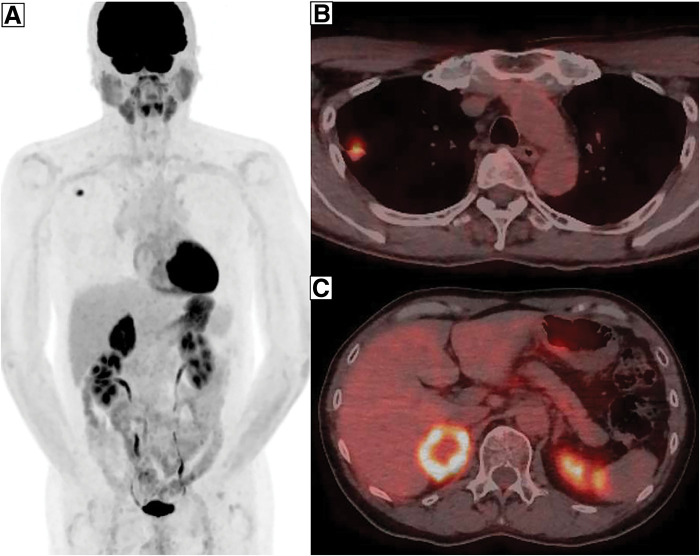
FDG-PET scans. FDG-PET scans show abnormal uptake (A) in the lung nodule (B, SUVmax = 7.2) and in the adrenal mass (C, SUVmax = 11.6). FDG, ^18^F-fluorodeoxyglucose; SUVmax, maximal value of standardized uptake value
